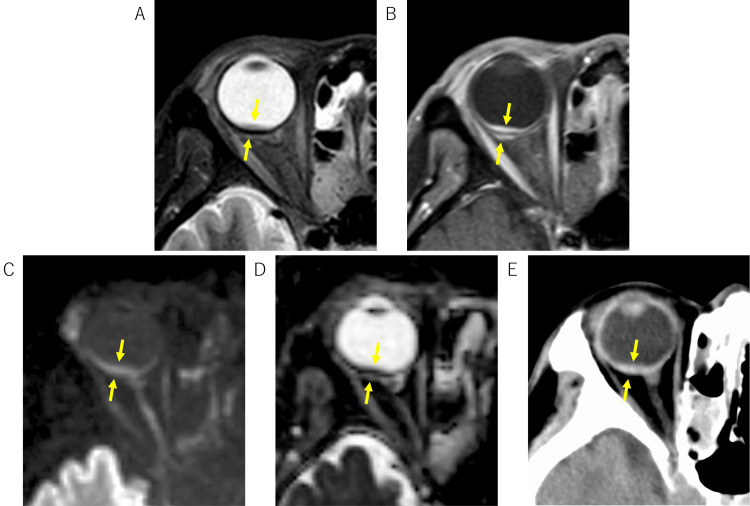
Intraocular findings on MRI and CT. Fat-saturated T2-weighted image shows wall thickening involving the inner and outer aspects of the posterior eyeball wall (A). Post-contrast T1-weighted image demonstrates uniform enhancement of the inner and outer aspects of the thickened wall (B). The lesion exhibits high signal intensity on diffusion-weighted imaging (C) and low apparent diffusion coefficient (D) values. CT shows high density in the corresponding area (E).
